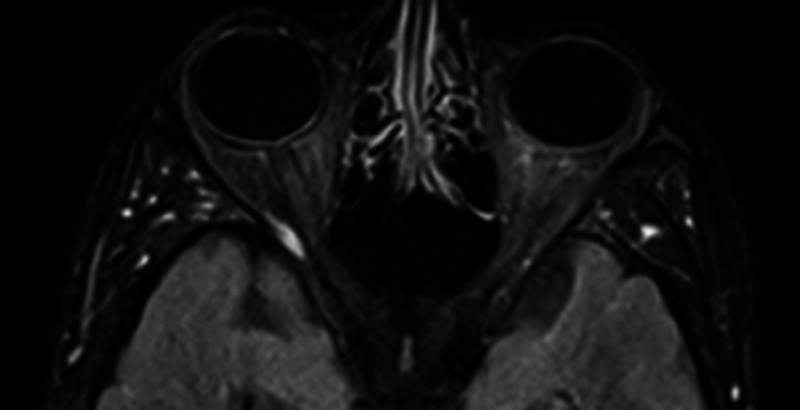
MRI brain fluid-attenuated inversion-recovery (FLAIR) post-contrast image showing normal both orbits and optic nerves, without any significant enhancement.
